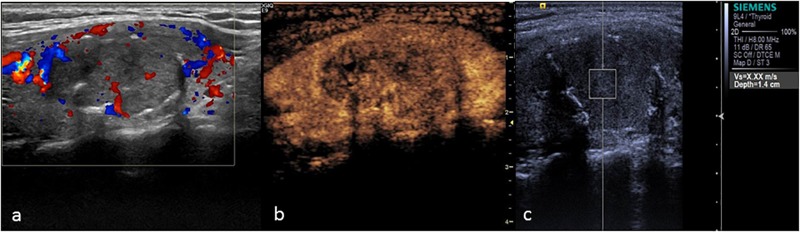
Nodular goiter. (a) Color Doppler ultrasound showing a hypo-echoic nodule with coarse calcification. (b) CEUS showing heterogeneous low enhancement. (c) The SWV value was X.XX m/s.
